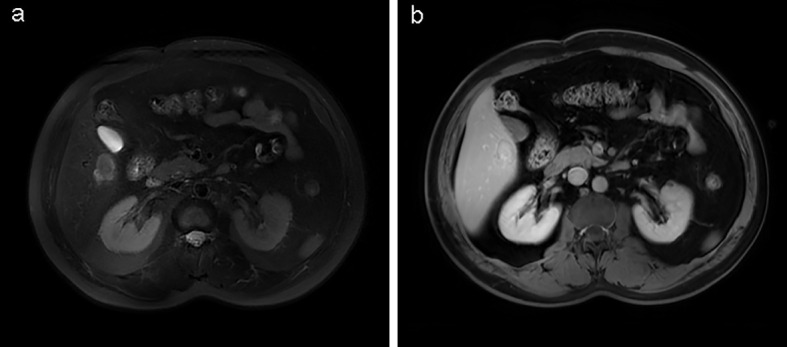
Liver MRI identified a lesion in the right posterior inferior hepatic segment, measuring approximately 2.2 × 3.1 cm. (a) T1-weighted imaging and (b) T2-weighted imaging revealed a lesion (white arrows) displaying slightly hypointense signal intensity on T1-weighted sequences and mixed hyperintensity on T2-weighted sequences.
